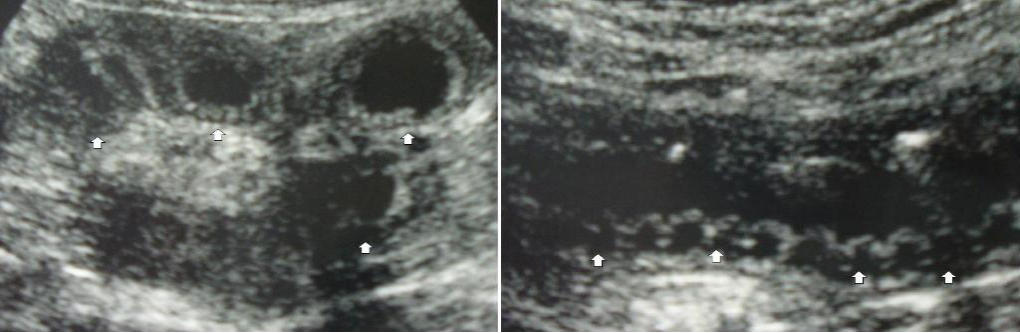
Ultrasonography of the abdomen. (A) Left image shows dilated bowel, diffuse bowel wall thickening (arrow). (B) Right image shows submucosal edema (arrow).
